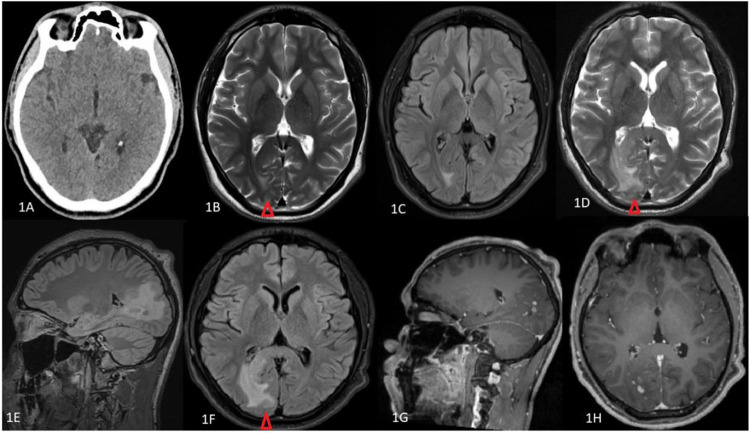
Radiological imaging. (A) CT of head without contrast (axial) does not show any abnormalities. (B and C) MRI of brain on 1st admission demonstrated small areas of right occipital subcortical patches of T2W (B) and FLAIR (C) bright signal. MRI of head on 2nd admission showed right parieto-occipital parasagittal subcortical and periventricular hyperintensity extending to the right side of splenium of corpus callosum on T2W axial (D), FLAIR sagittal (E) and FLAIR axial (F) sections (red arrowhead). MRI of head with contrast sagittal (G) and axial (H) sections revealing patchy nodular branching pattern of post-contrast enhancement of the hyperintense areas on T2/FLAIR. The enhancement is seen extending to the ependymal surface of occipital horn of right lateral ventricle.
